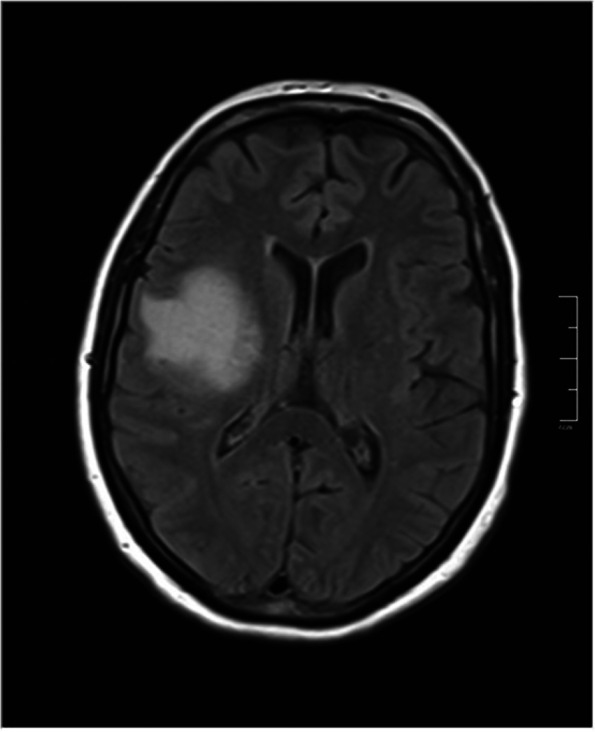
Second brain MRI (FLAIR sequence) showed hyper-intense lesion involving periventricular and subcortical white matter
